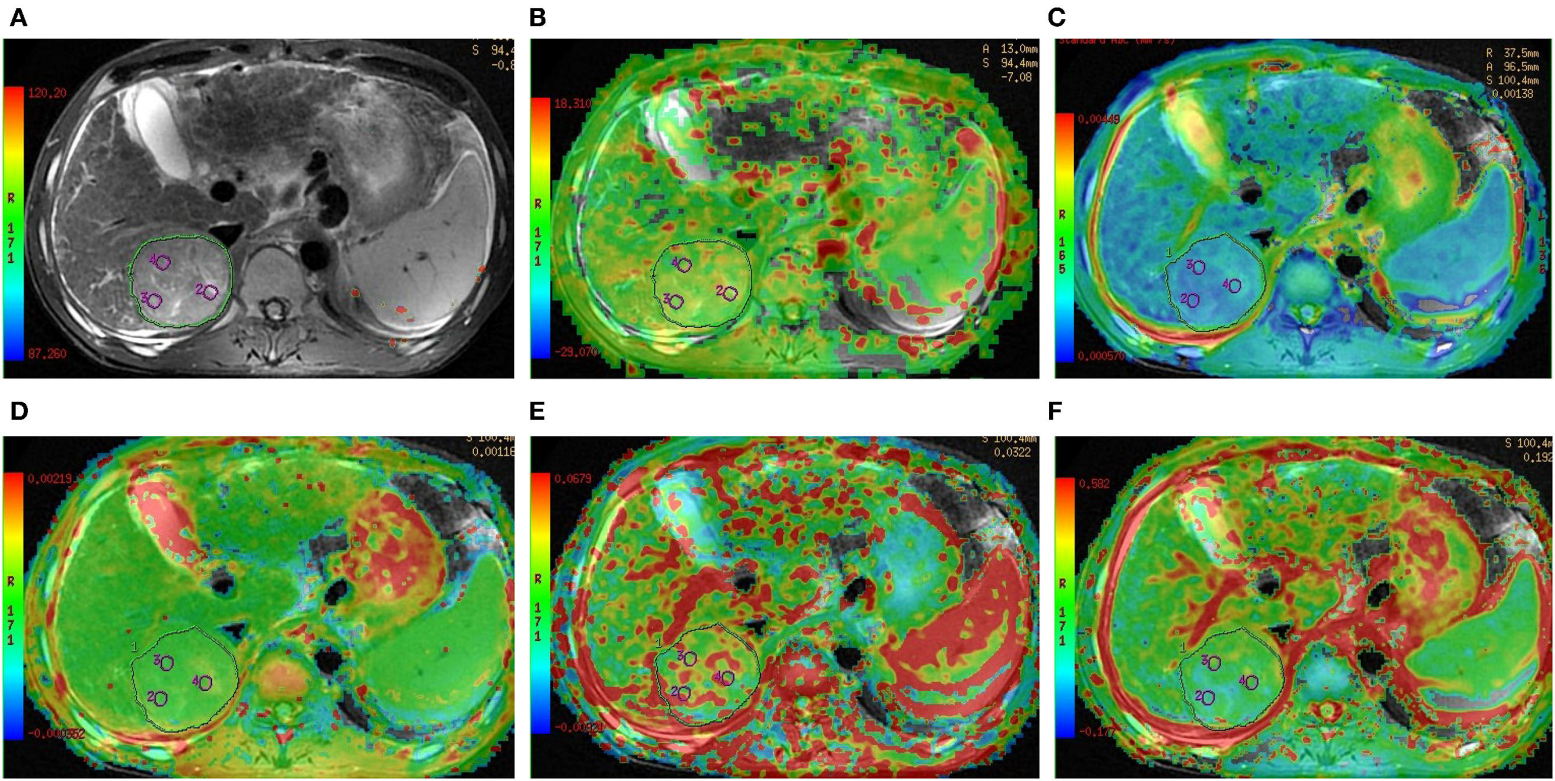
Preoperative MR images of one lesion in a 52-year-old man with grade 2 HCC. (A) T_2_-weighted image, (B) APT image of HCC fused with T_2_-weighted imaging indicates that the average APT SI value determined by two observers is 3.17%, (C–F) IVIM-derived pseudo-colored maps of HCC (ADC, D, D*, and *f* , respectively) fused with T_2_-weighted imaging indicate that the average ADC, D, D*, and *f* values determined by two observers were 1.54 × 10^−3^ mm^2^/s, 1.59 × 10^−3^ mm^2^/s, 100.18 × 10^−3^ mm^2^/s, and 34.55%, respectively.
